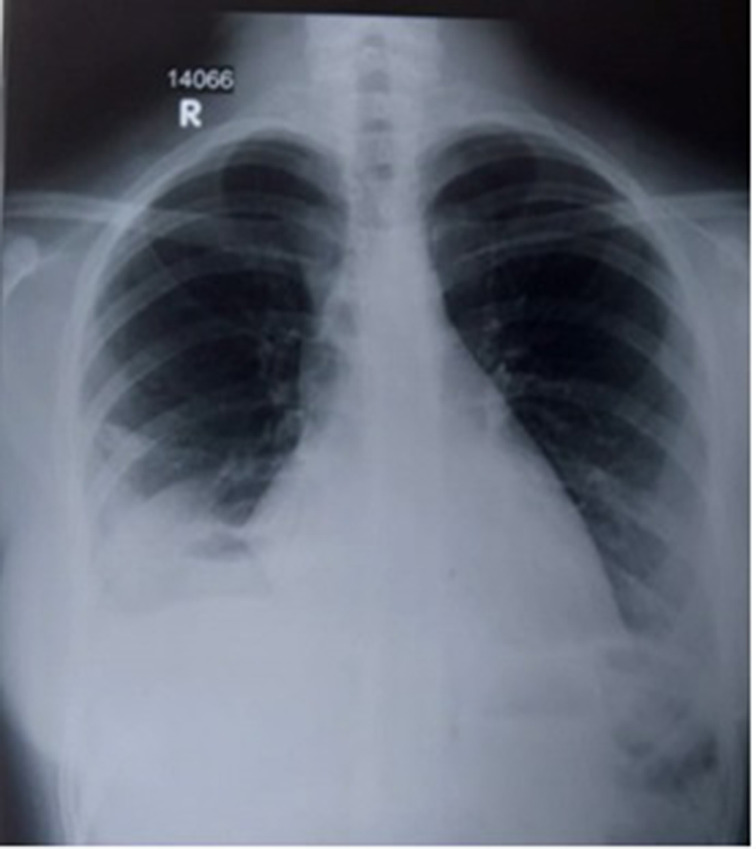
Post-aspiration chest radiograph.
